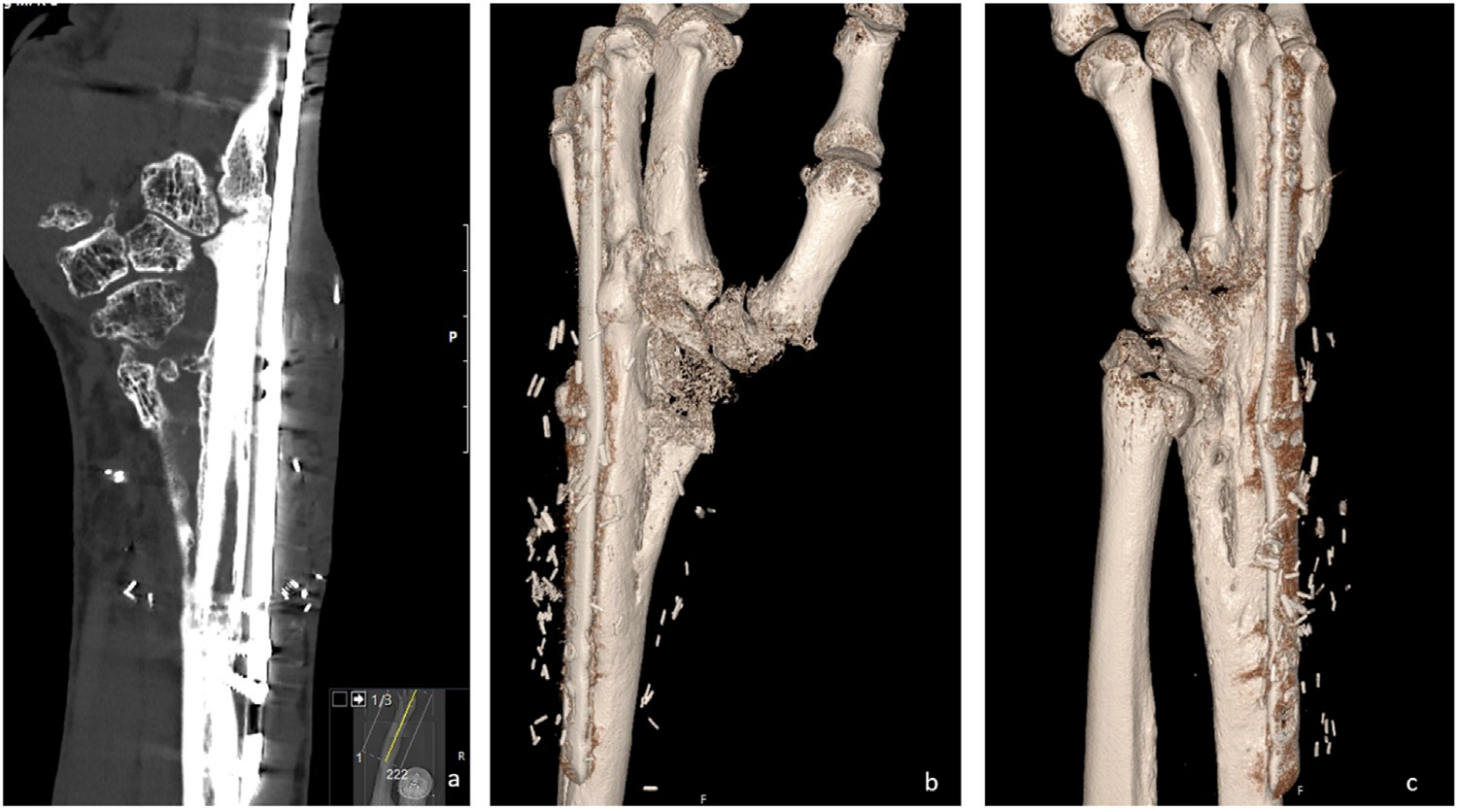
after Fibula transfer a: CT 12 month after the procedure b and c: 3D CT 12 month after the procedure.
